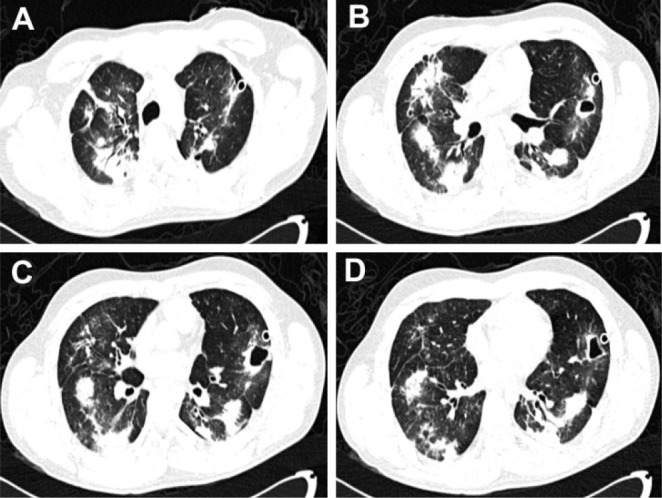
Chest CT - Scan of patient.
